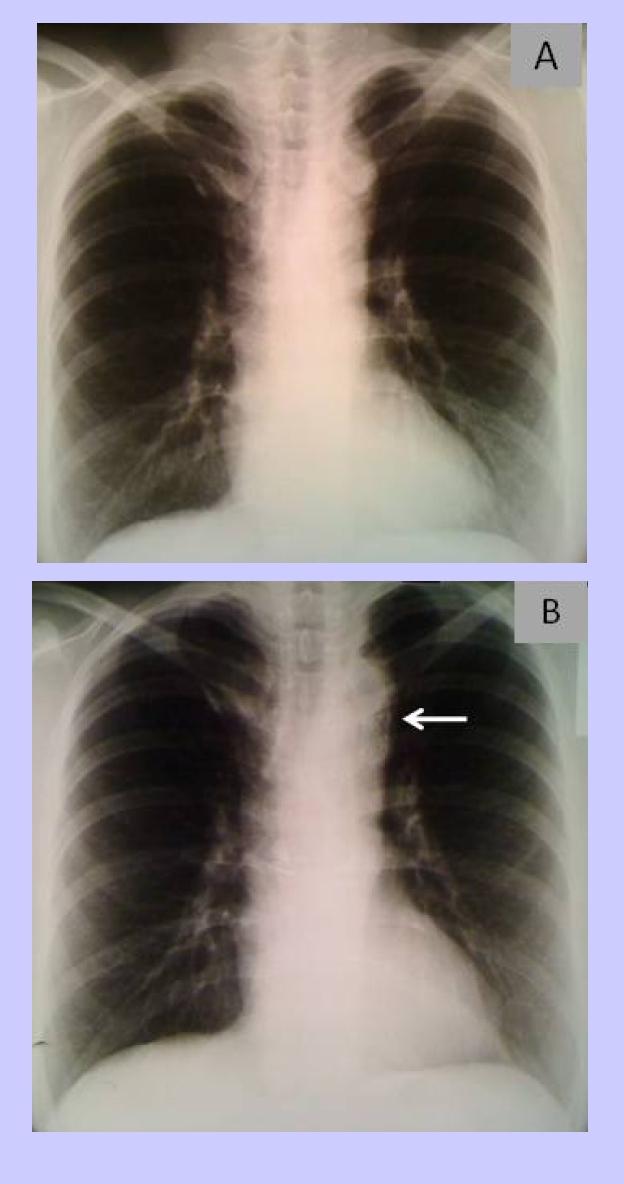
Chest radiography. A Before stent placement and B  After stent placement (see arrow for stent position)
